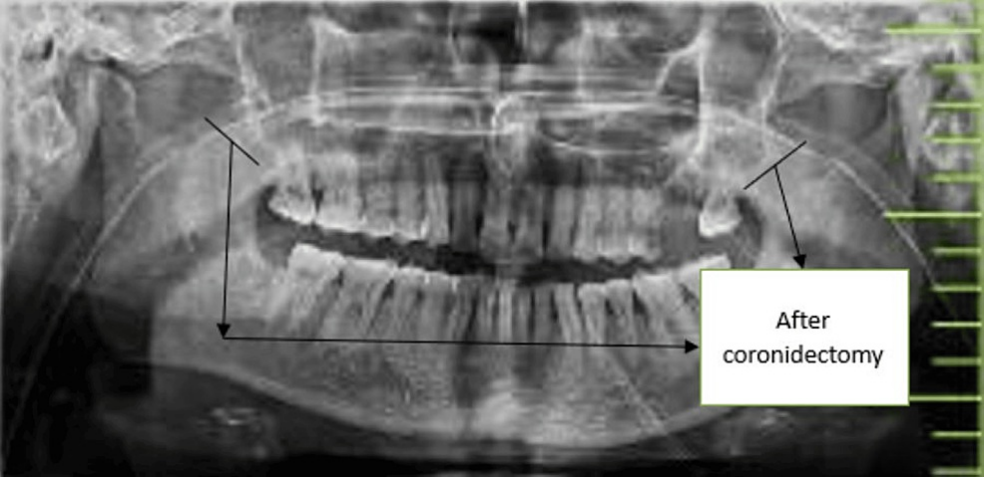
Post-operative orthopantomography showing reduction in coronoidectomy
